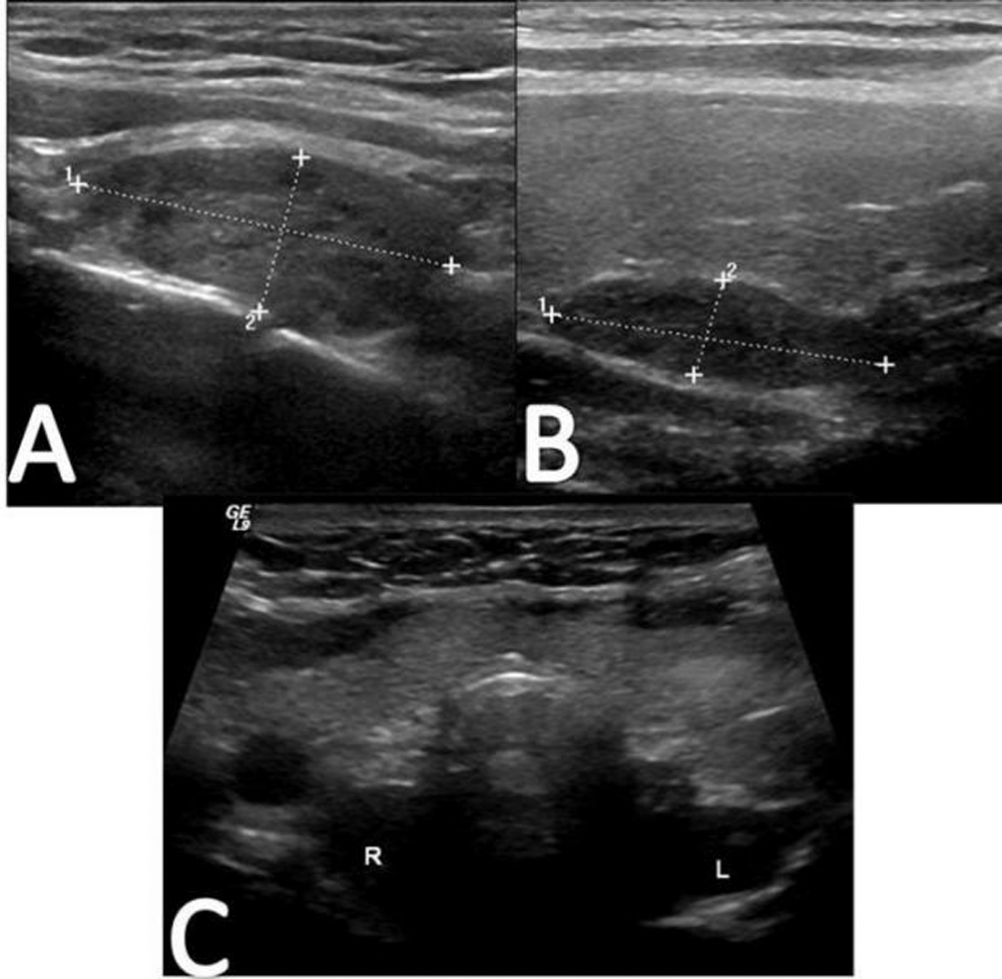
Representative images of the ultrasound of the thyroid and parathyroid glands are shown. (A) demonstrates a sagittal view of the right lobe of the thyroid, and a mass measuring 2.93 × 1.23 cm is demarcated by lines 1 and 2 is noted posteriorly in the tracheoesophageal groove. (B) demonstrates a 2.63 × 0.69 cm mass in tracheoesophageal groove posterior to the left lobe of the thyroid. (C) shows a transverse view of the entire thyroid gland, with the hypoechoic masses noted posteriorly (R,L).
